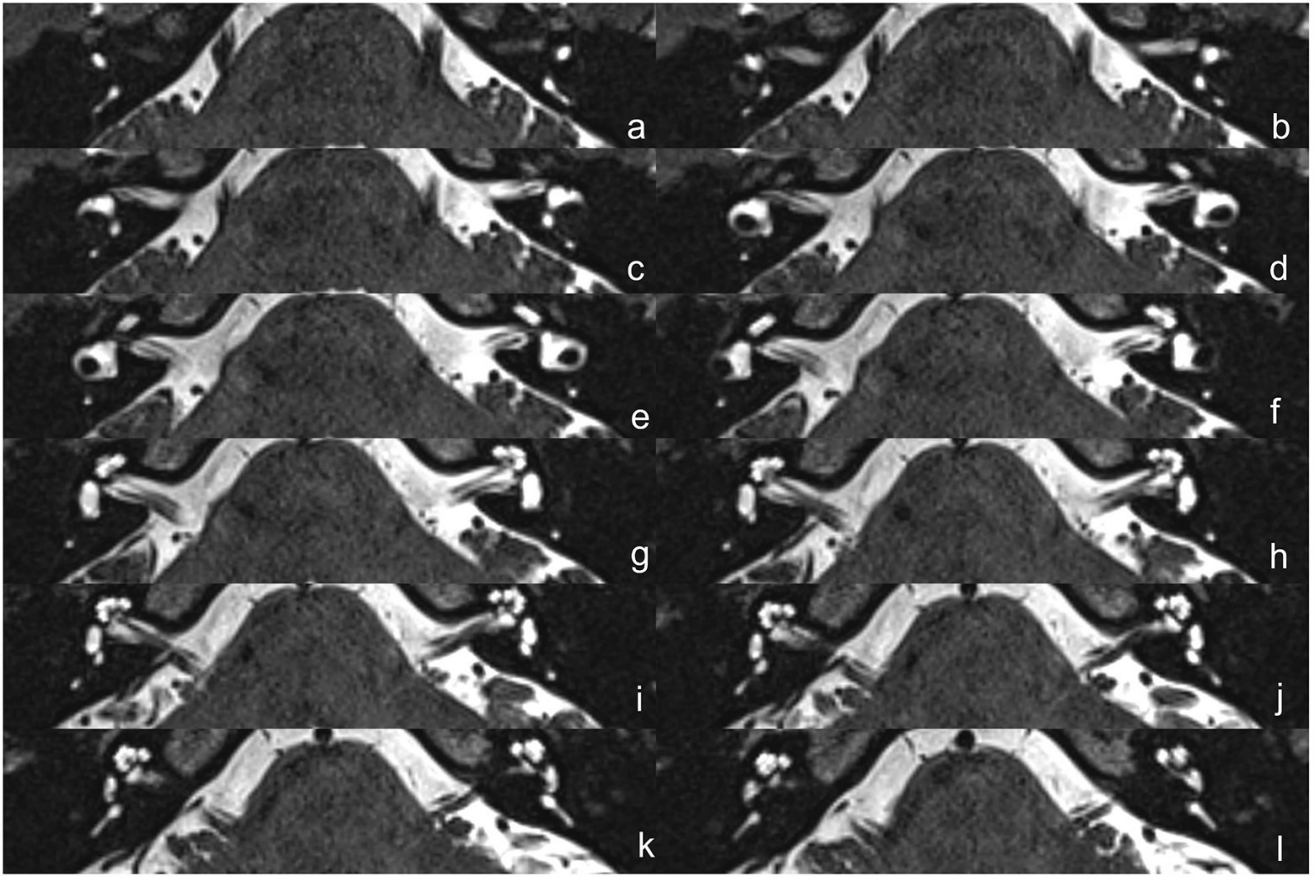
The 3D-SPACE MRI images of a 48-year-old female with VM. (a–l) Axial, high-resolution, and T2-weighted MRI scan showing non-visualization of the vestibular aqueduct on both sides. *3D-SPACE*, three-dimensional sampling perfection with application optimized contrasts using different flip angle evolutions, *VM*, vestibular migraine.
